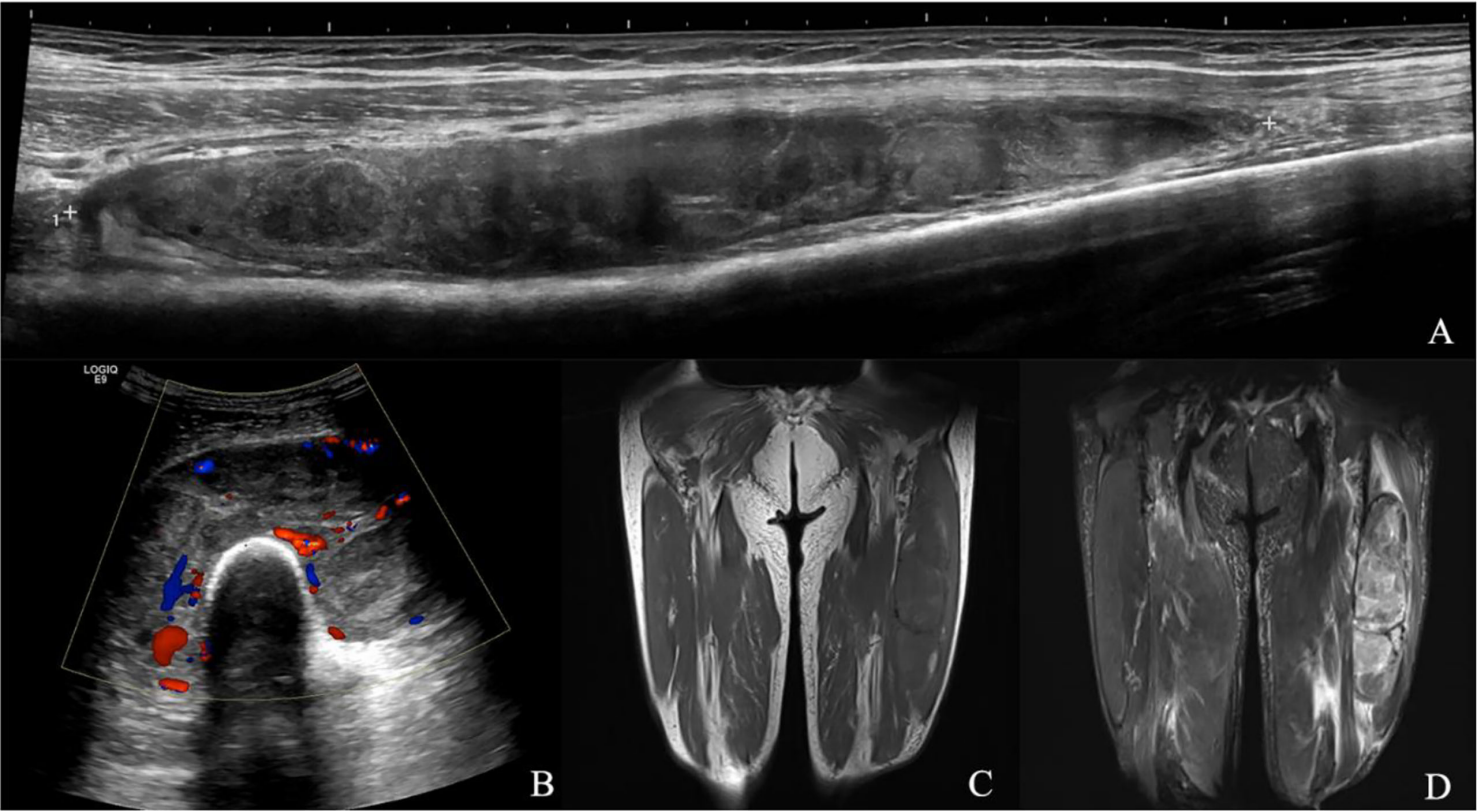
Myxofibrosarcoma of lower extremity. A 68-year-old male presented with a swelling in his left lower leg. (A) Longitudinal grayscale US showed a well-defined, partial unsmooth, heterogenous intramuscular mass with a maximum diameter of 201 mm in the intermediate vastus muscle. (B) Color Doppler imaging of the same area showed hypervascularity within the tumor, corresponding to type IV. (C) Coronal T_1_WI showed a heterogeneous intensity mass. (D) The lesion had relatively heterogeneous high SI on the coronal fat suppressed T_2_WI with maximum diameter of 278 mm and microlobulated margin. (A, B) A score of 6 was assigned, indicating a malignant tumor (true positive). (C, D) A score of 3 was assigned, indicating a malignant tumor (true positive).
